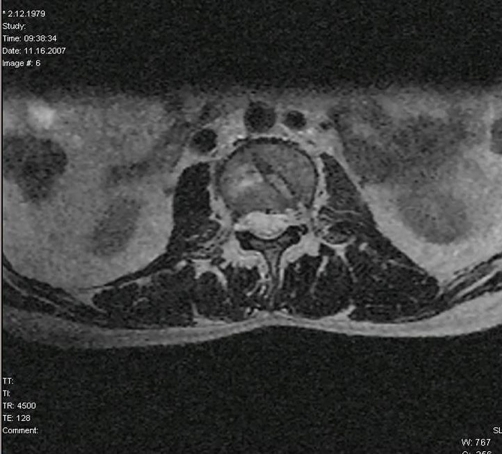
Axial MRI imaging shows mild compression of the spine cord.
